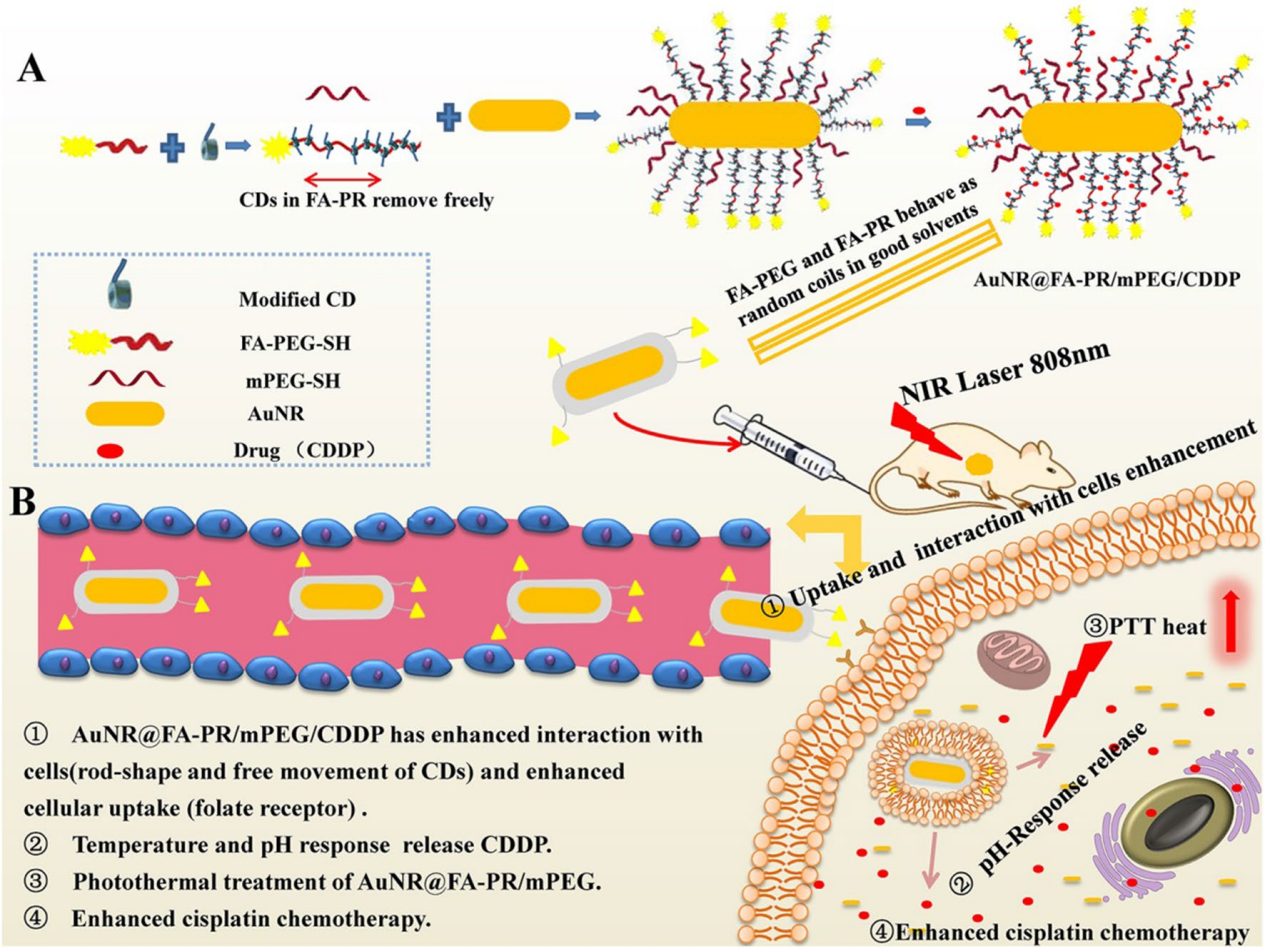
A Preparation of AuNR@FA-PR/PEG/CDDP; B Mechanism of photothermal therapy (PTT)/chemotherapy (CT) synergistic treatment of cancer with AuNR@FA-PR/PEG/CDDP
